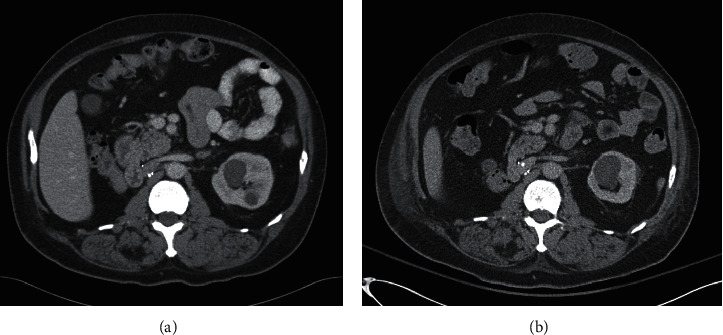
Contrast-enhanced CT scan of the abdomen (a) two years before transplant and (b) five months after transplant. (a) Left kidney showing a 3.1 × 2.6 cm, nonenhancing, cystic lesion at the upper pole. (b) Enlarged and minimally enhancing 3.3 × 3.8 cm mass in the upper pole of the left kidney suspicious for renal cell cancer (white arrow).
